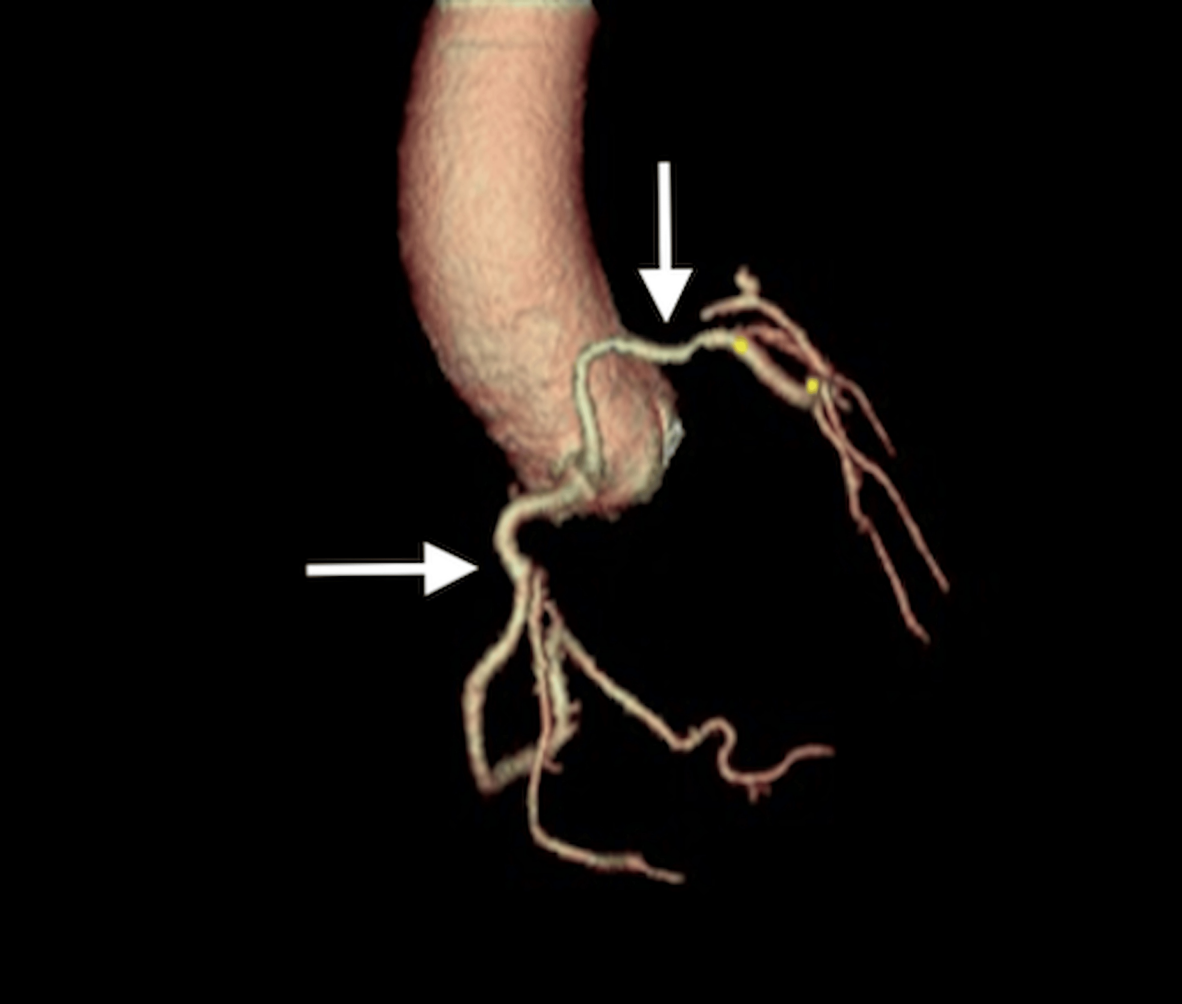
3D coronary computed tomography angiography Three-dimensional coronary computed tomography angiography (CTA) demonstrating anomalous origin of the left main coronary artery (LMCA). The LMCA (vertical arrow) arises from the right sinus of Valsalva (RSV) via a shared ostium with the right coronary artery (RCA) (horizontal arrow). The 3D reconstruction clearly depicts the single coronary origin from the RSV prior to the bifurcation of the LMCA into its major branches.
